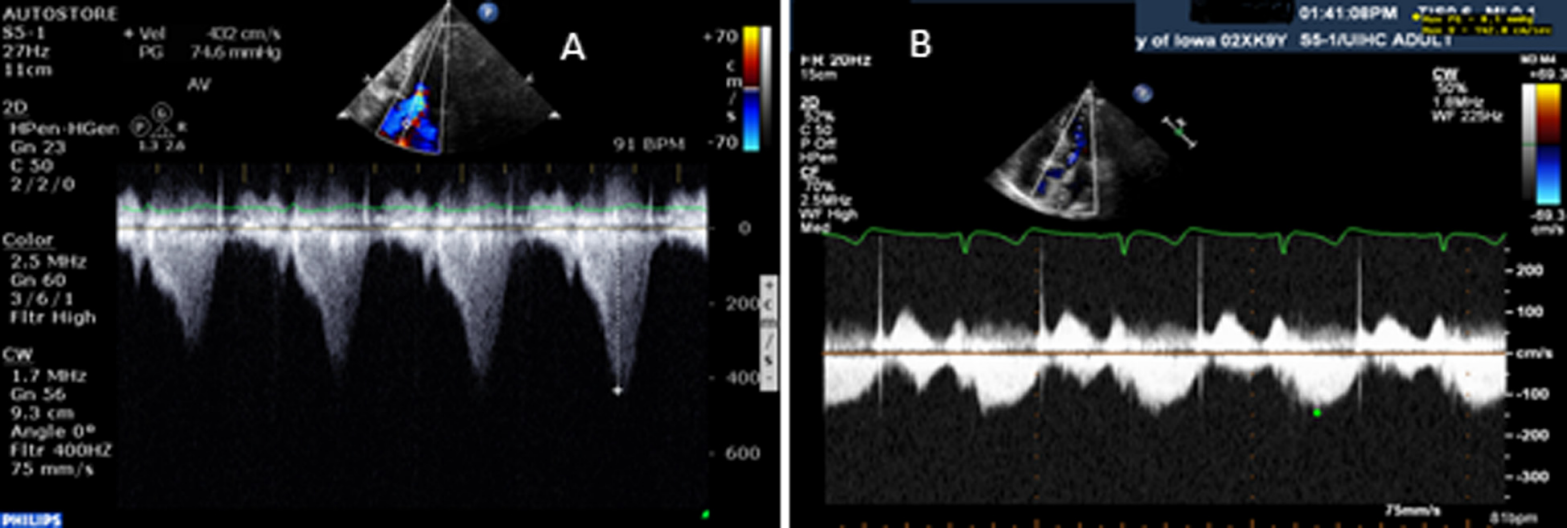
Continuous-wave Doppler through the left ventricular outflow tract. (A) Continuous wave Doppler through the left ventricular outflow tract - immediate postoperative period. (B) Continuous-wave Doppler through the left ventricular outflow tract - postoperative day 5.
